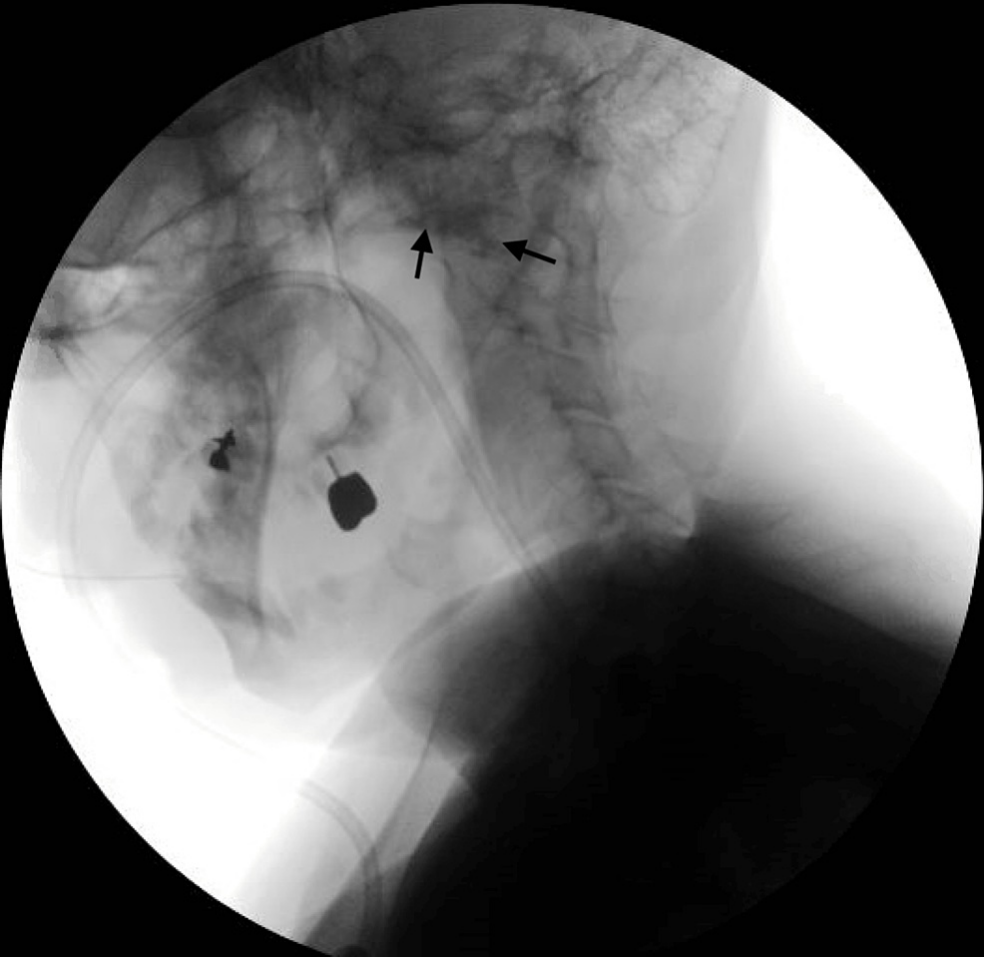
X-ray swallow function with fluoroscopy showing residue present within the valleculae and piriform sinuses.
